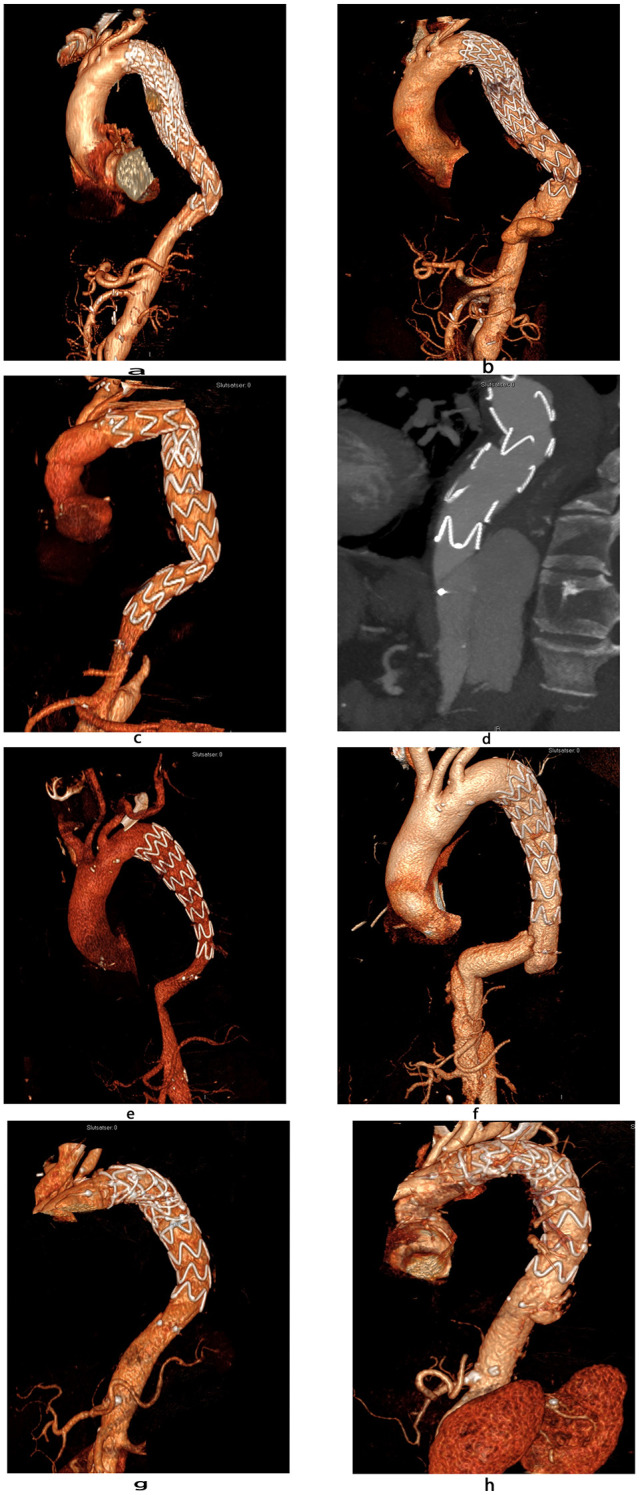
Three-dimensional multi-planar reconstruction of postoperative computed tomography scans of all cases with the development of a distal stent-graft induced new entry after treatment with a dissection-specific stent-graft.
